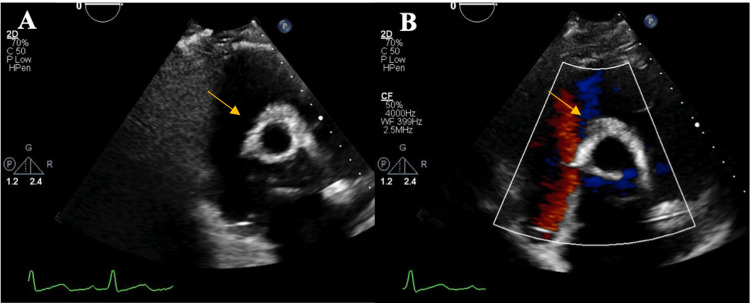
Post-operative transthoracic echocardiogram demonstrating bioprosthetic aortic valve. (A) After the patient underwent replacement of the aortic valve and root replacement and repair of the right atrial wall, follow-up transthoracic echocardiography demonstrated a well-seated bioprosthetic aortic valve (yellow arrow) without valvular or paravalvular regurgitation. (B) Color Doppler showing no persistent aorto-atrial fistula.
